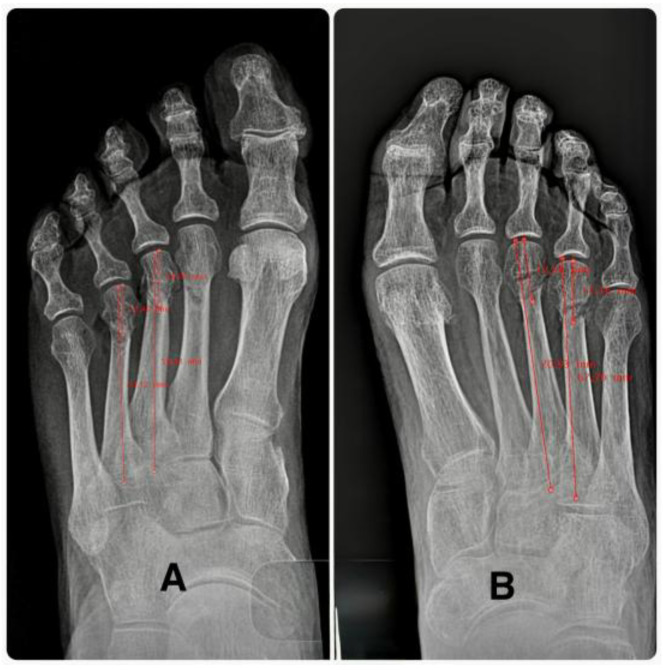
(A): Xray measurements before the osteotomy. Length of 3rd and 4th metatarsals (mm) (B). Osteotomy measurement. Distance from the osteotomy to the articular line (mm).
